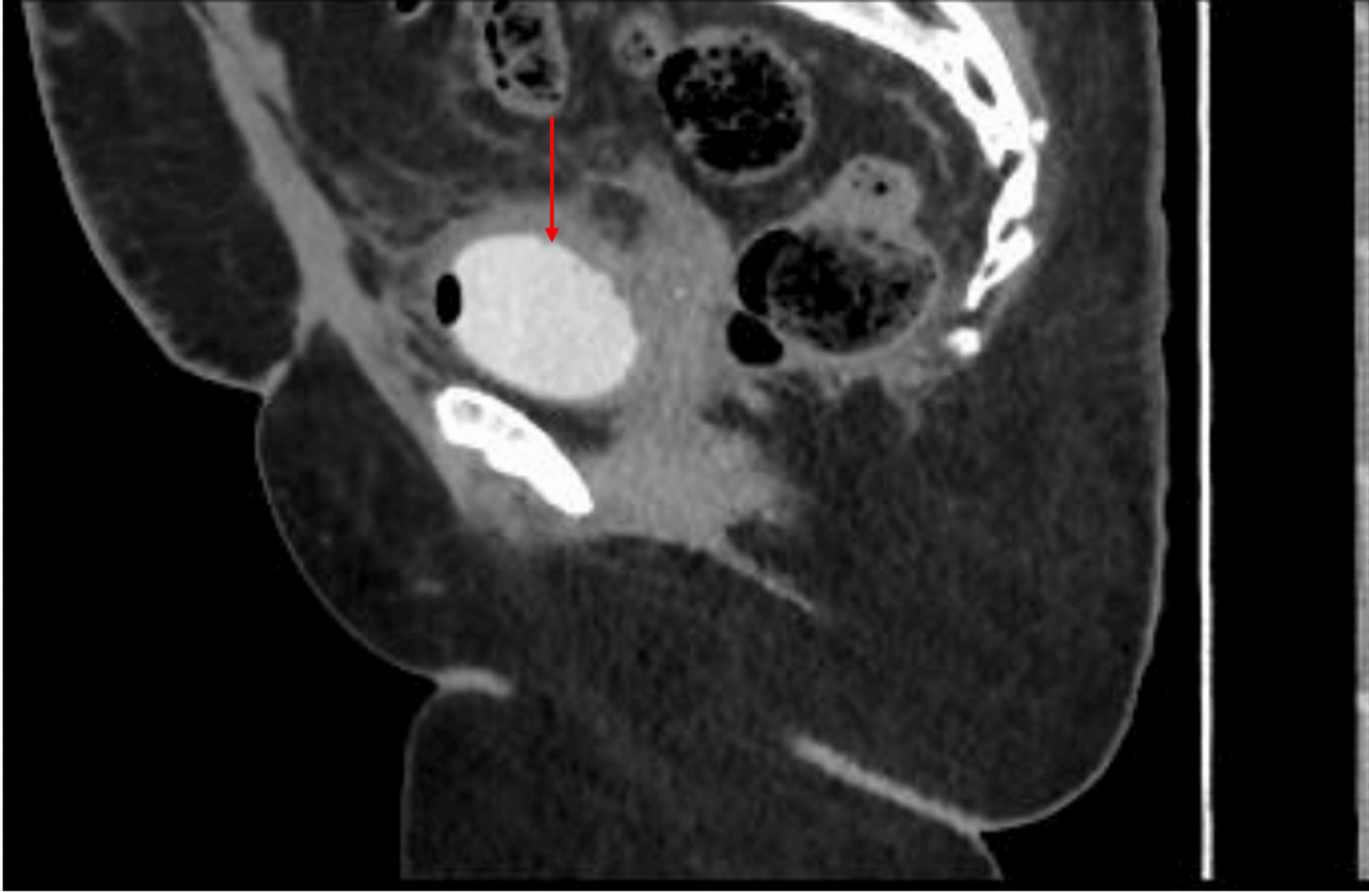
Postoperative CT cystogram with contrast. The red arrow is pointing at the bladder.
